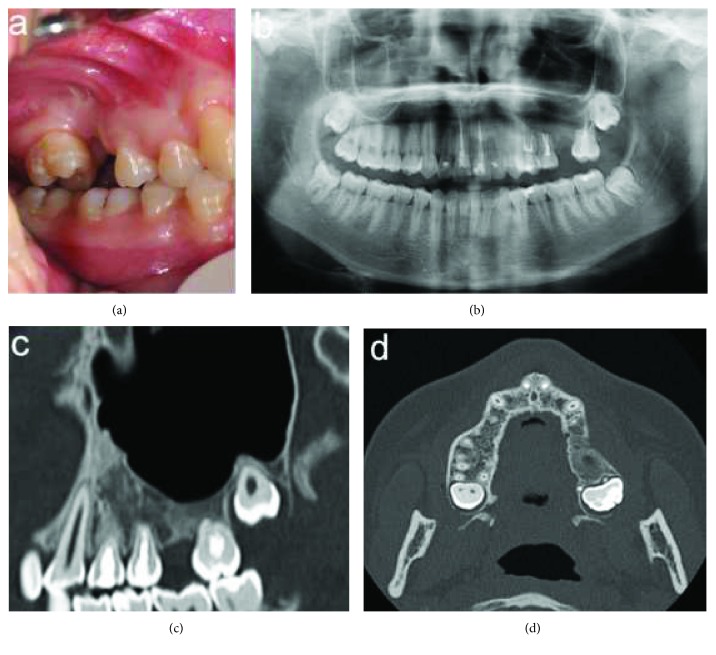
Twelve-month follow-up: (a) intraoral view, (b) ortopantomography, (c) CT sagittal view, and (d) CT axial view.
